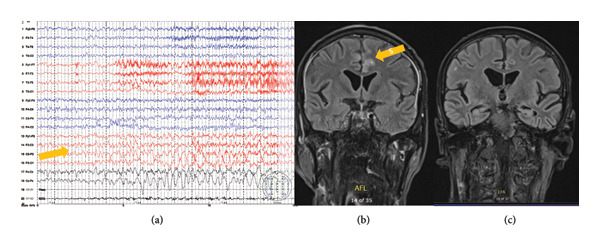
(a) Standard EEG confirmed subclinical focal seizures from the left centroparietal region, (b) brain MRI showed a T2w/FLAIR hyperintense lesion in the superior frontal and cingulate gyrus bilaterally, and (c) follow‐up brain MRI on T2w/FLAIR showed regression of previously identified hyperintense lesions.
